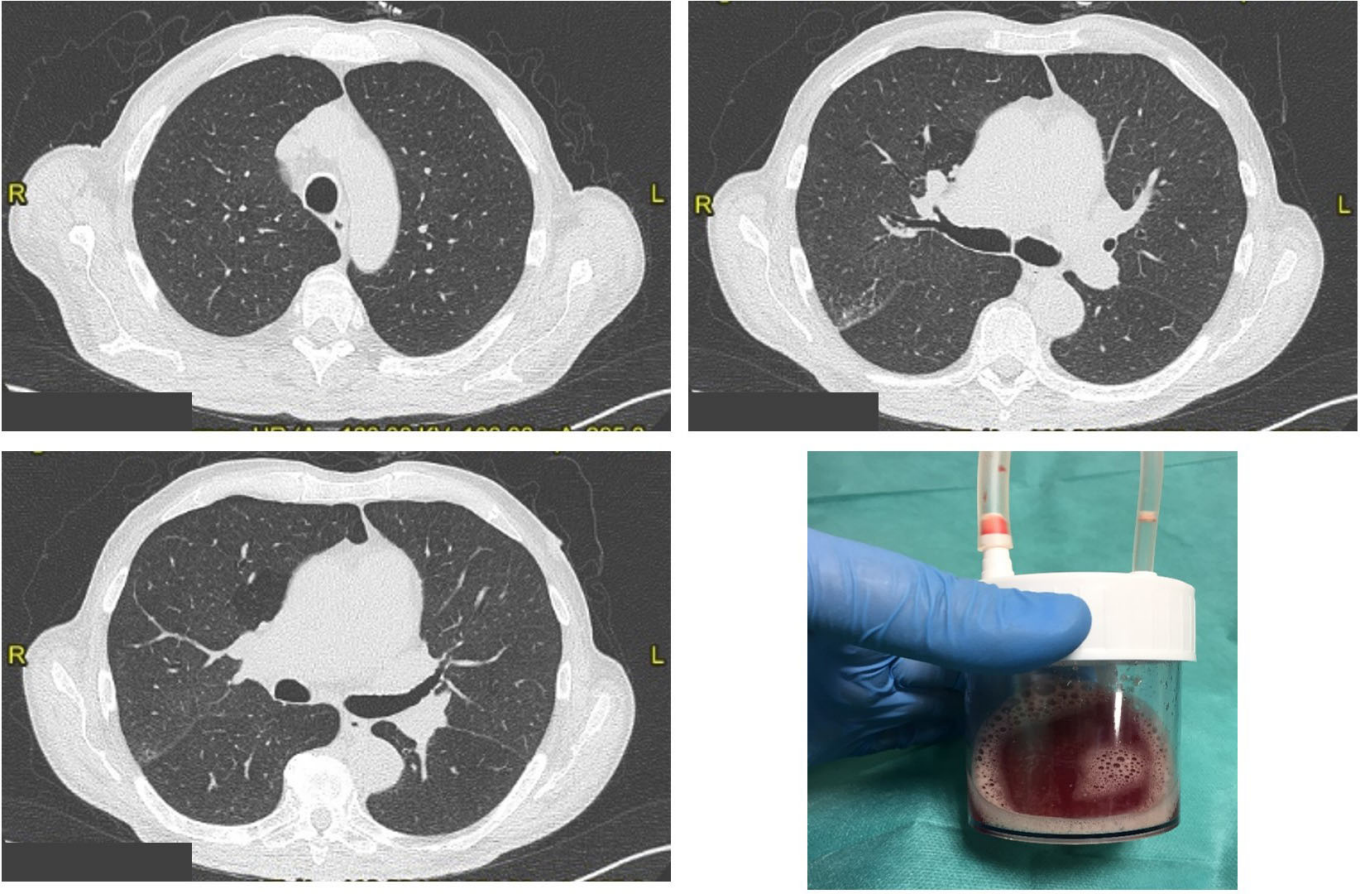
Chest computed tomography scan after resolution of diffuse alveolar haemorrhage and the bronchoalveolar lavage confirming the presence of blood.
